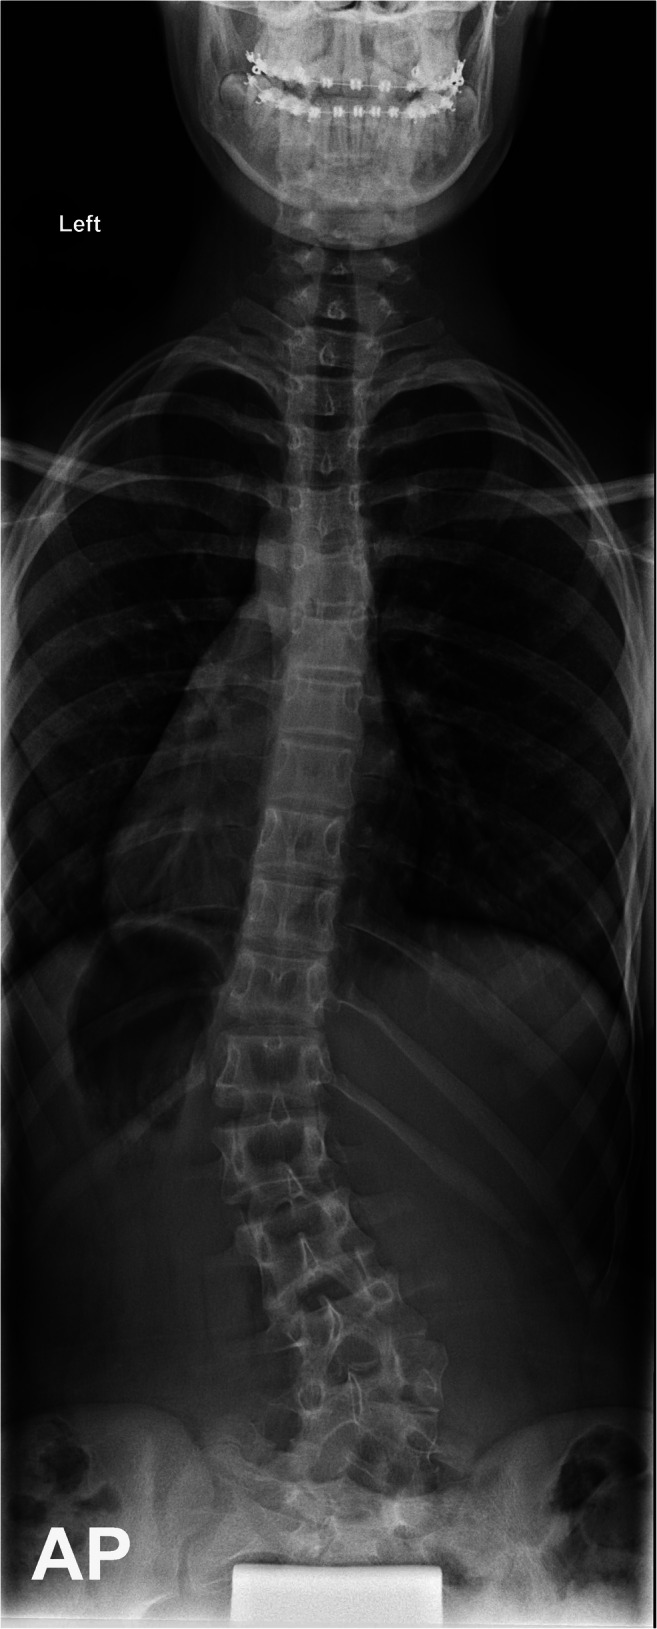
Preoperative imaging. Total spine x-ray in ap view (standing)
